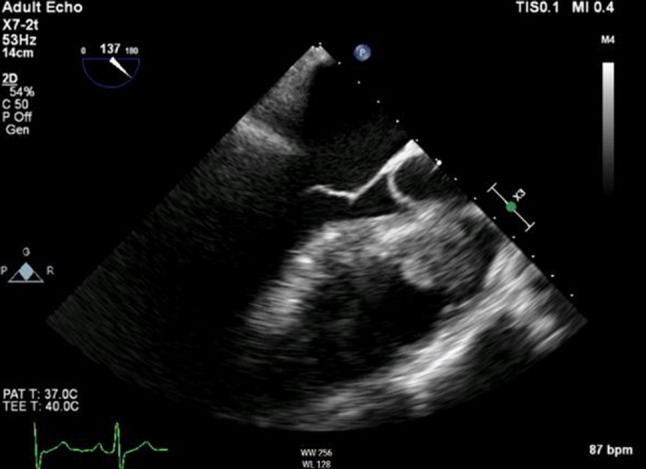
TEE showing thickened pulmonary valve and atrial septal defect. *TEE* transoesophageal echocardiography
